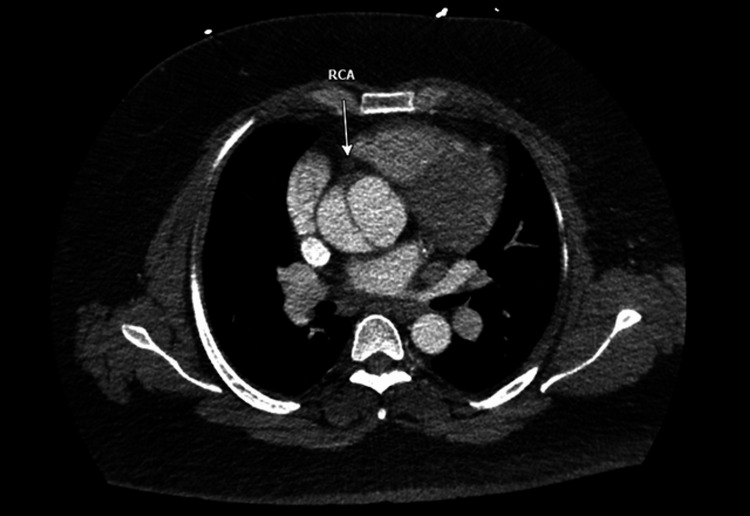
Chest computed tomography angiography showing a dissection flap occluding the ostium of the right coronary artery and lack of opacification of the right coronary artery with contrast as highlighted by the white arrow RCA: right coronary artery
